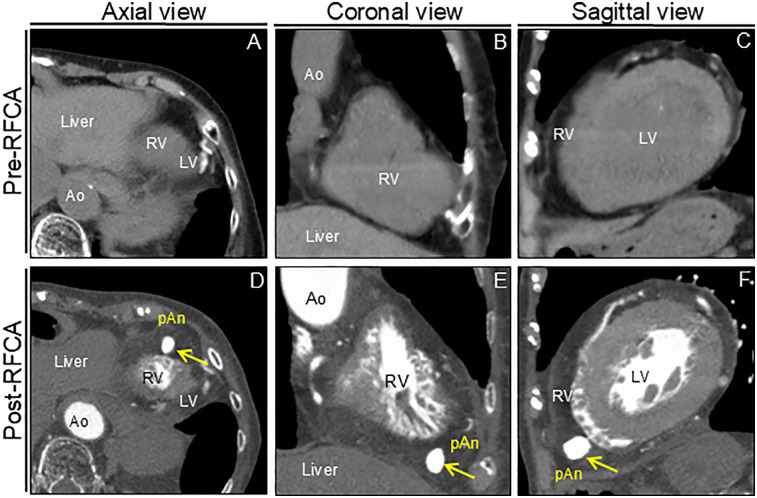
Computed tomography images before and after RFCA. Preprocedural unenhanced computed tomography images confirm normal right ventricular anatomy (A–C). Postprocedural cardiac computed tomography angiography images reveal apical outpouching of the right ventricle (arrows) (D–F). Ao, aorta; LV, left ventricle; pAn; pseudoaneurysm; RFCA; radiofrequency catheter ablation; RV, right ventricle.
